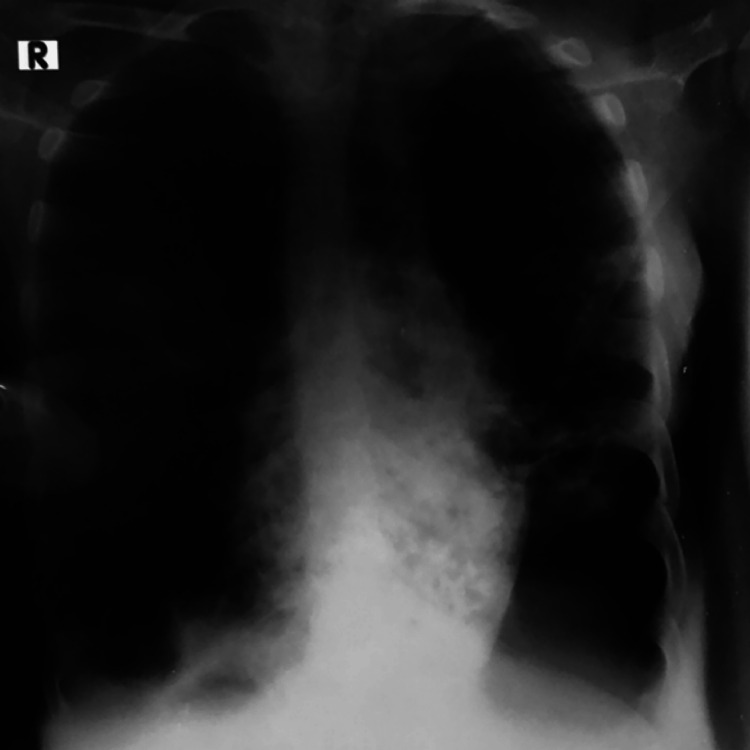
Chest x-ray of the patient showing bilateral bronchiectasis with cyst in the left lower zone and hyperinflated lower lung field
